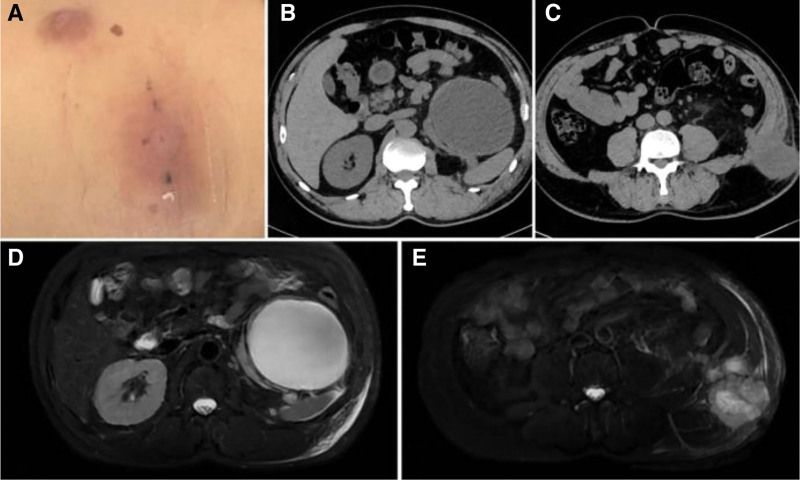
(A) Appearance of skin mass: redness around 2 sites of the original laparoscopic ventral incision on the left abdominal wall, hard texture beneath the incision; (B, C) preoperative contrast-enhanced CT: cystic low-density shadow in the left kidney measuring approximately 10.7 × 9.5 cm, with clear boundaries and localized dense shadows at the margin; multiple masses with heterogeneous density posterior to the left kidney and in the left posterior abdominal wall, the largest measuring approximately 4.3 × 4.8 cm; abdominal wall lesions involving the left latissimus dorsi, internal oblique, and transversus abdominis muscles; (D, E) preoperative MRI appearance: oval-shaped cystic lesion in the left kidney showing non-enhancing long T2/long T1 signal, clear boundaries; multiple masses and nodular lesions with long T2/long T1 signal in the subcutaneous tissue of the left posterior abdominal wall, hyperintense on DWI, irregular in morphology.
